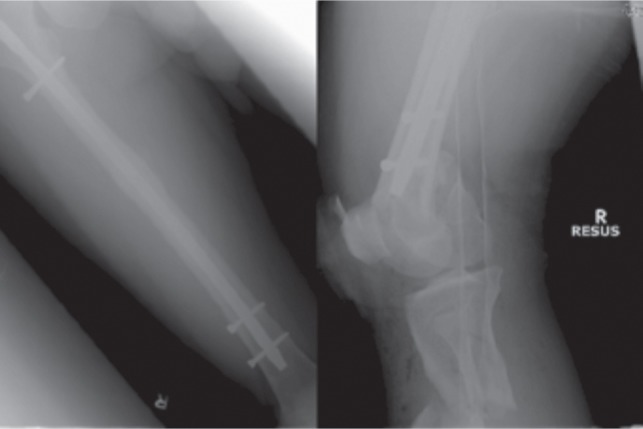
(i) Radiograph after intramedullary nail insertion. (ii) Radiograph right distal femur after polytrauma.
